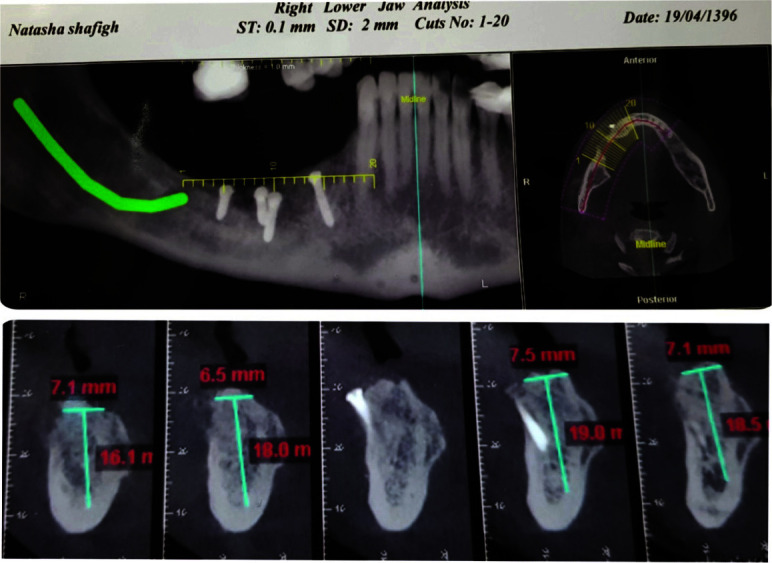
Cone-beam computed tomography (CBCT) after reconstruction with iliac bone graft
